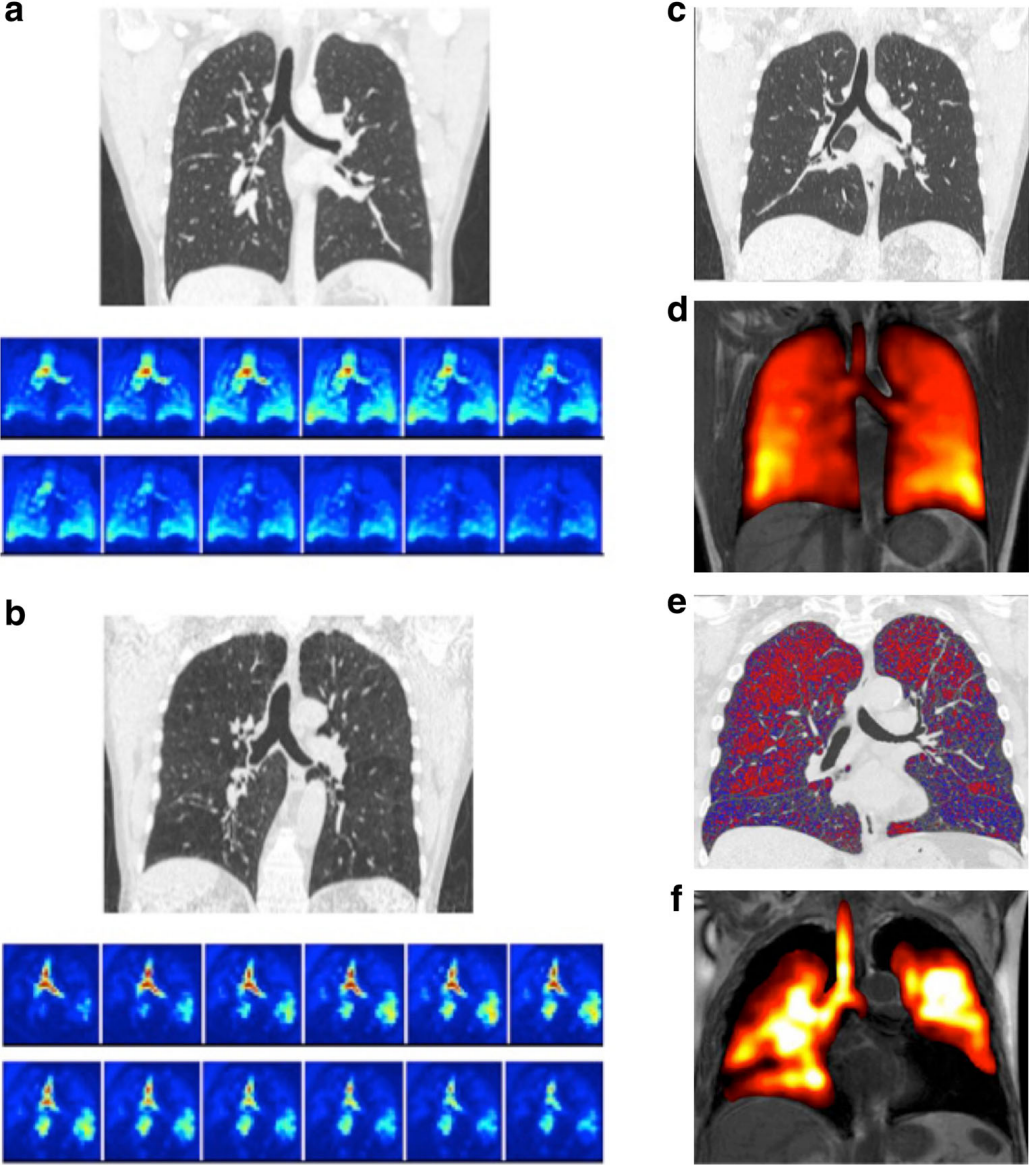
Dynamic pulmonary ventilation in (a) a healthy participant and (b) COPD Patient 1. The top and bottom subfigures in (a) and (b) show the QCT in lung window and temporal change in ventilation level during a natural breathing cycle, respectively. Note the marked ventilation defects demonstrated in the COPD patient. c CT of the participant in (a). d Fused DXeV-MRI and ^1^H-MRI for (a). e CT with highlighted emphysematous changes for the patient in (b). (f) Fused DXeV-MRI and ^1^H-MRI for patient in (b)
